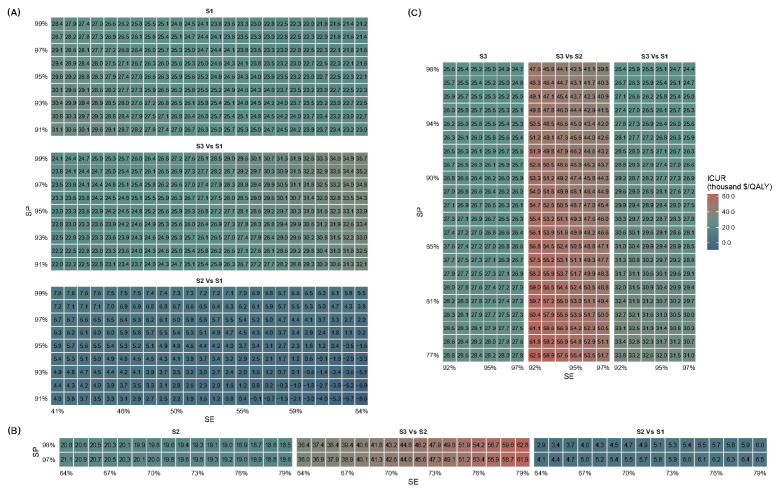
Dominance maps showing optimal strategies across sensitivity and specificity for (A) ultrasound, (B) FibroScan^®^, and (C) MRI-PDFF. Note: SE, sensitivity; SP, specificity; S1, WHtR + ultrasound strategy; S2, WHtR + FibroScan^®^ strategy; S3, WHtR + MRI-PDFF strategy; ICUR, incremental cost–utility ratios; MRI-PDFF, magnetic resonance imaging-proton density fat fraction. The results show that S2 consistently dominated S1, while S3 was optimal only at higher WTP thresholds or with high diagnostic performance.
